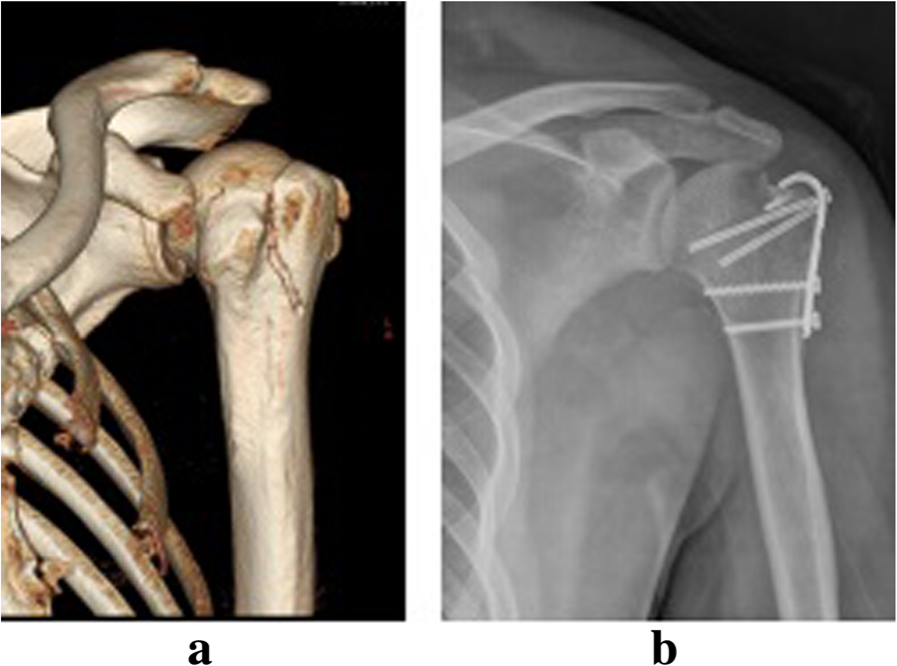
The radiographs of patients with avulsion fractures of greater tuberosity of humerus treated with hook plate fixation. a Preoperative posteroanterior X-ray films; (b) Posteroanterior X-ray films after 3 months postoperatively
